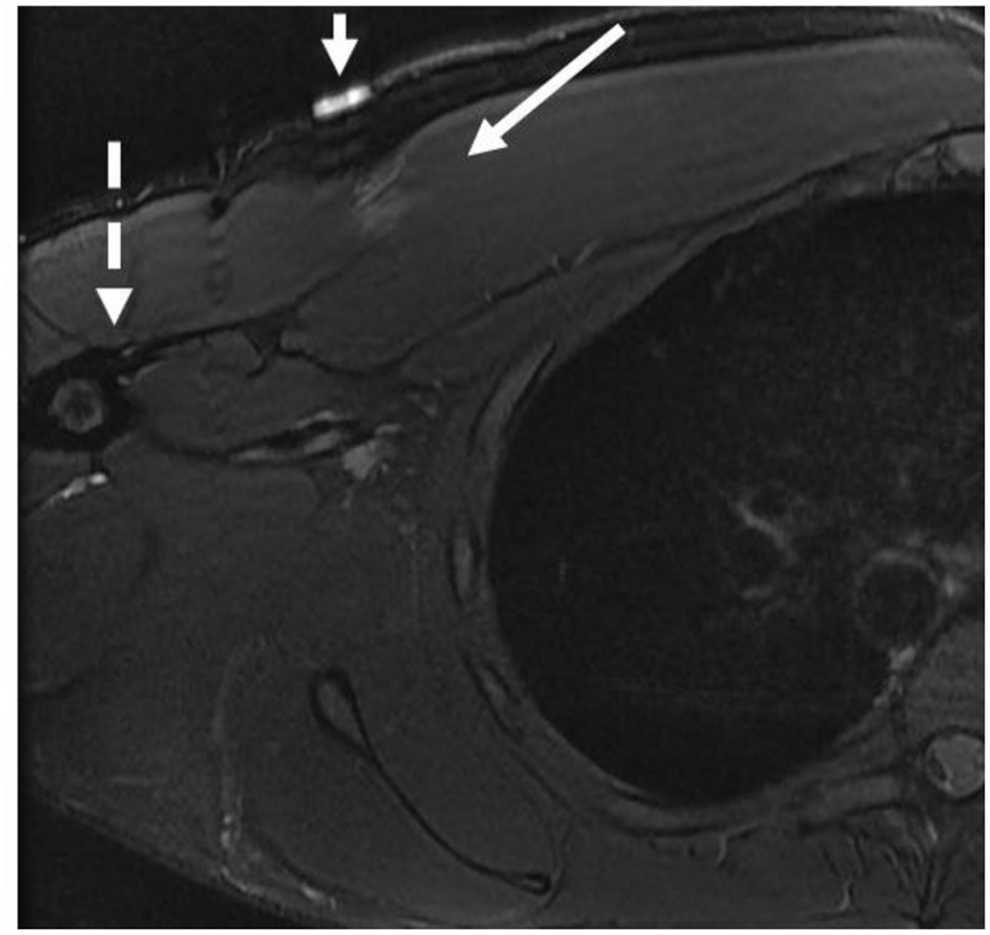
24-year-old-male with left anterolateral chest pain underlying the marker (short arrow). Axial T2-weighted with fat-saturated MR image shows feathery edema-like signal (long arrow) underlying the marker at the myotendinous junction of the sternal head of the pectoralis major muscle related to low grade (grade I) strain. Note that the distal tendon is intact (dashed arrow)
